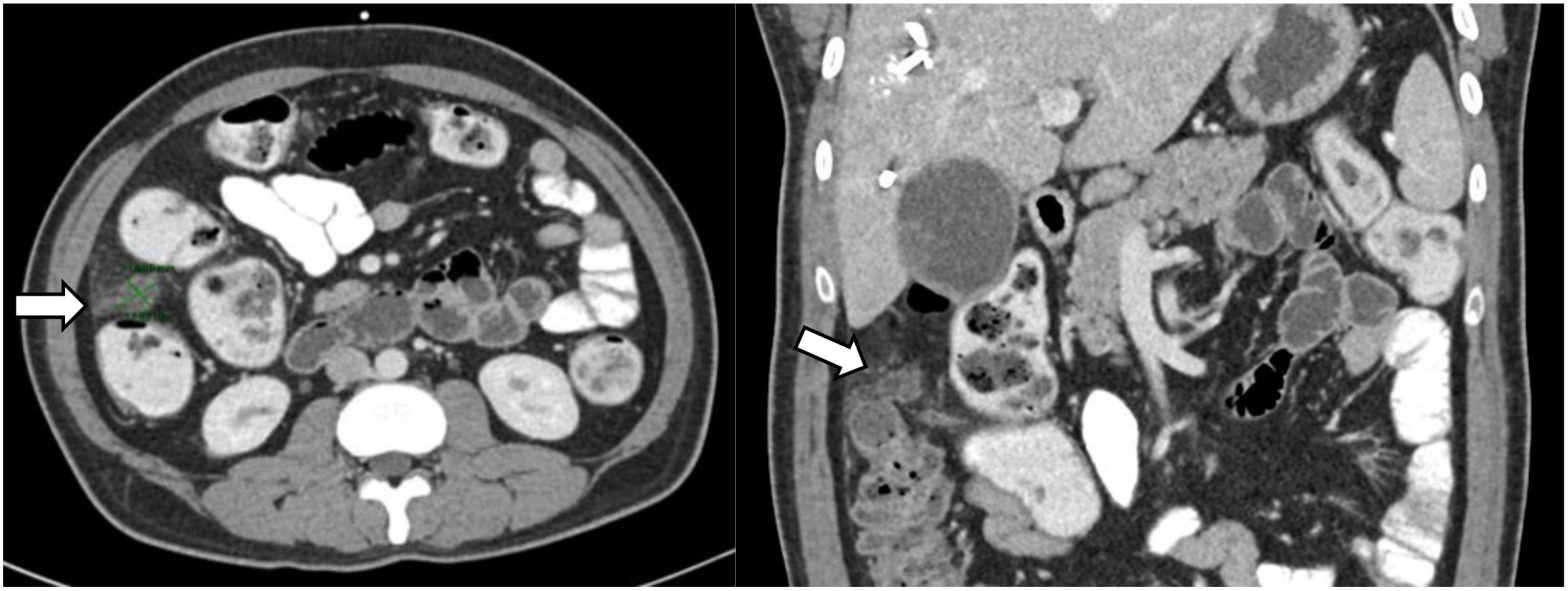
Interval CT scan after right portal vein embolisation demonstrating new 2-centimetre intra-peritoneal soft tissue lesion (white arrow), suspicious for peritoneal metastasis—this was eventually confirmed on diagnostic laparoscopy and biopsy.
